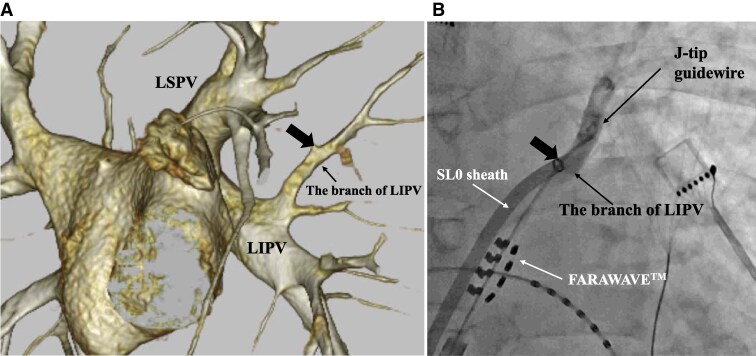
(*A*) Three-dimensional computed tomography showing the left inferior pulmonary vein, where the guidewire subsequently became entrapped (arrow). (*B*) Selective venography showing the J-tip guidewire entrapment within the small branch of the left inferior pulmonary vein (arrow). LIPV, left inferior pulmonary vein; LSPV, left superior pulmonary vein.
